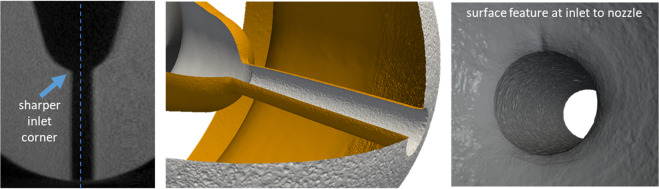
A surface representation derived from high-resolution X-ray computed tomography of the fuel injector (ECN Spray C37) used in this study reveals an asymmetrically drilled hole and a 20 μm wide ridge on the nozzle wall at the inlet. Slice from synchrotron X-ray CT scan (left), full iso-surface (middle), zoom-in view of inlet to nozzle (right). Images produced with data from Matusik *et al*.^1^.
